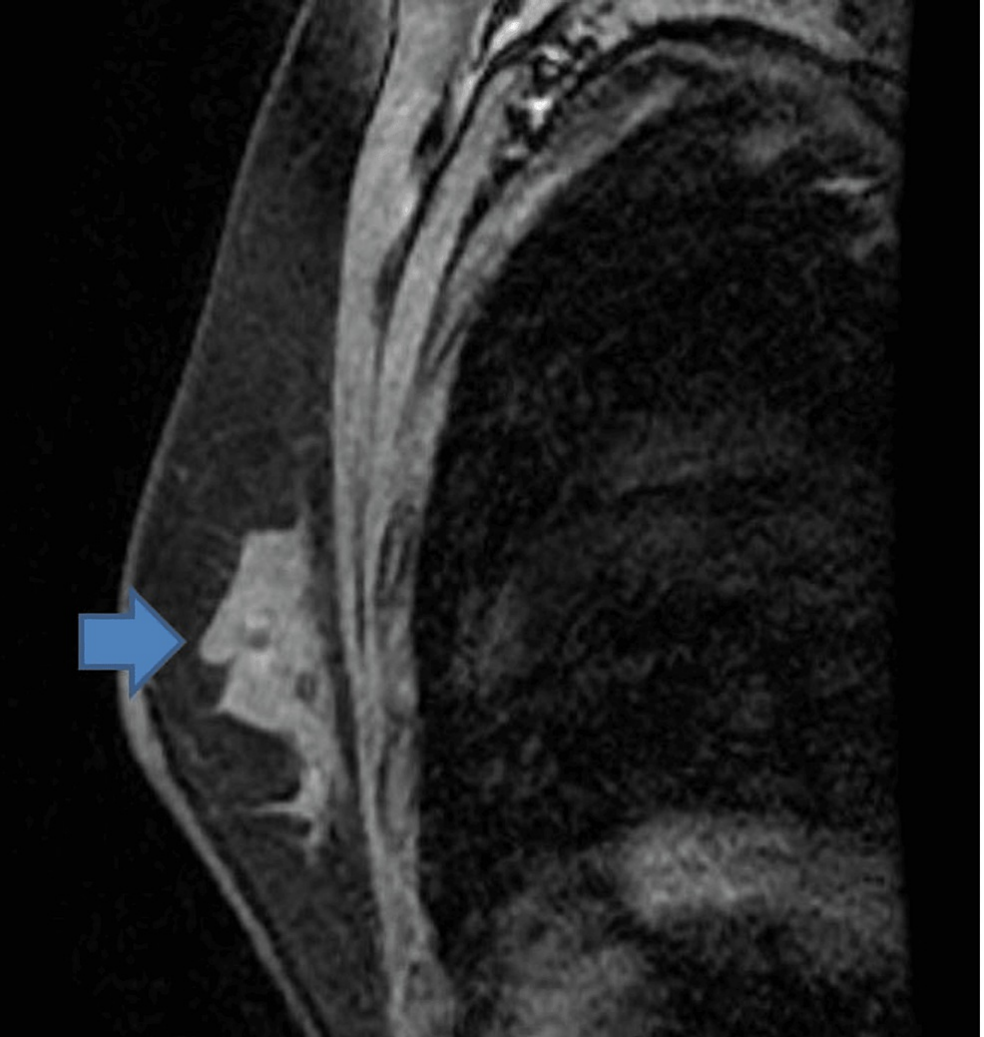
Follow-up MRI showing an irregular left breast mass (blue arrow).
